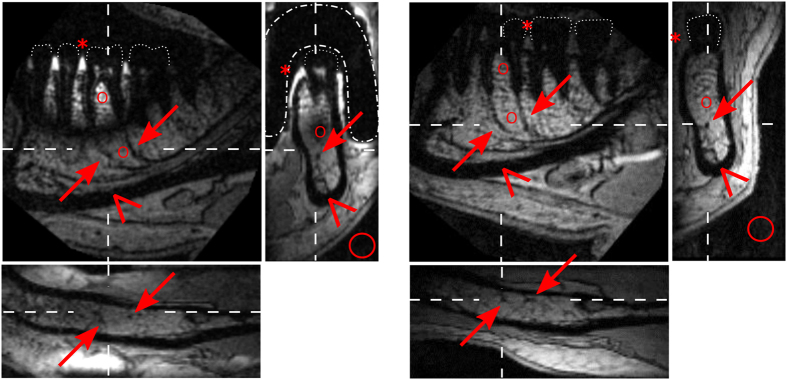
In-vivo* dental MRI of a mandible acquired with (a–c) and without (d–f) intraoral coil C_3_ in conjunction with a 4-cm loop coil. Orthogonal slices were reconstructed from a 3D-FLASH MRI that was acquired in 3:57 min at 3 T at a resolution of 250∙250∙500 μm^3^. Note that the intraoral coil enhanced the signal of the interdental gingiva (asterisk) and pulp strongly and caused a signal hypointensity at the roots. Both configurations nicely depicted the inferior alveolar nerve and its branches to the apices of the teeth (rami dentales) as indicated by arrows and wedges, respectively. Dashed lines indicate the position of the reconstructed slices, dotted lines the crowns of the teeth, dash-dot lines the position of the intraoral coil. The regions where the signal and noise was measured are indicated by asterisks (gingiva) and circles, see Table 2.
